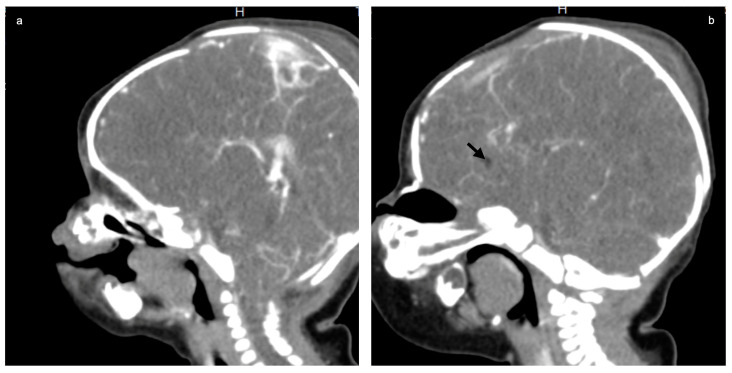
Brain contrast CT (sagittal MPR): agenesis of the corpus callosum (a) with a small anterior midline lipoma (b); black allow and small posterior fossa with cerebellar tonsillar ectopia, the tonsils extending below the level of the foramen magnum (a).
